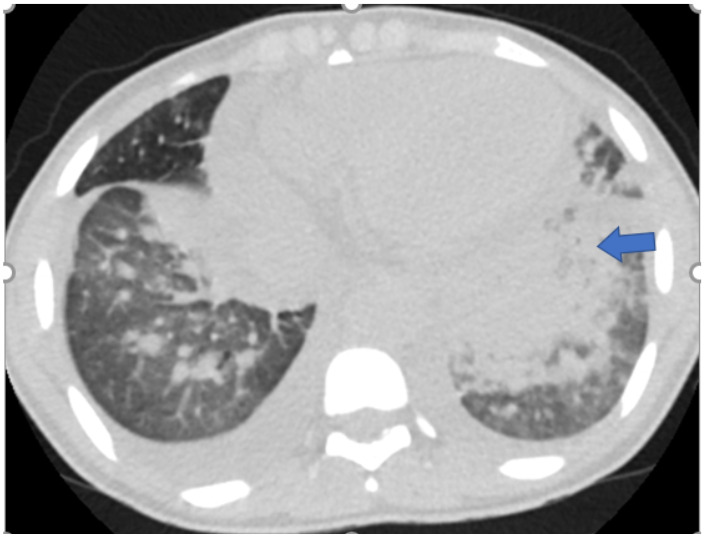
An axial non-contrast enhanced chest CT scan; lung window of a 66-year-old woman with fever and breathlessness for 9-days shows diffuse, bilateral, posterior basal, ground glass infiltrates, dense consolidation (Arrow) and air bronchograms with minimal right pleural effusion. Typical features. RT-PCR confirmed COVID-19.
